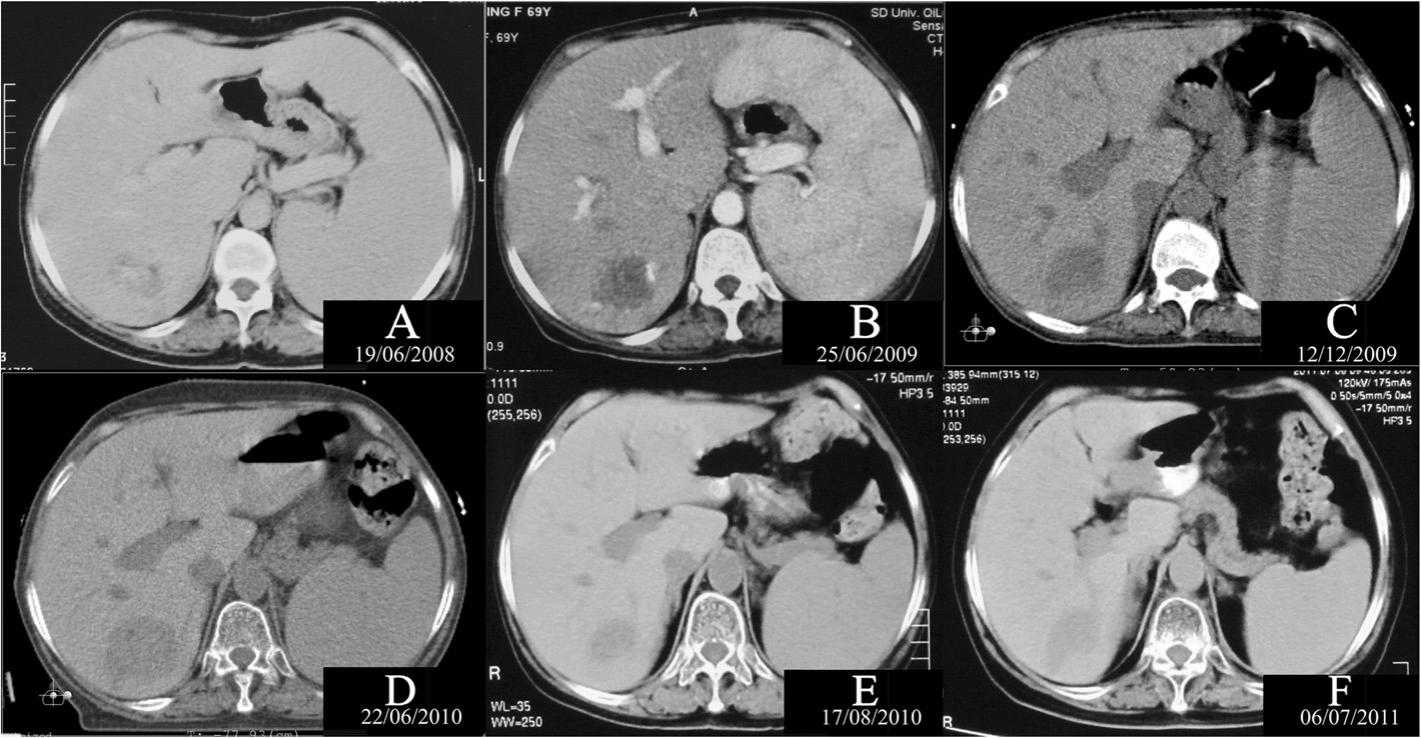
Sequential computed tomography scan images at the same level. A, At the diagnosis of the disease (19/06/2008); B, Relapsed after chlorambucil and thalidomide (25/06/2009); C, Before the first stage of 3D-CRT (12/12/2009); D, Before the second stage of 3D-CRT (22/06/2010); E, At one month after the second stage of 3D-CRT (17/08/2010); F, At one year after the second stage of 3D-CRT (06/07/2011).
